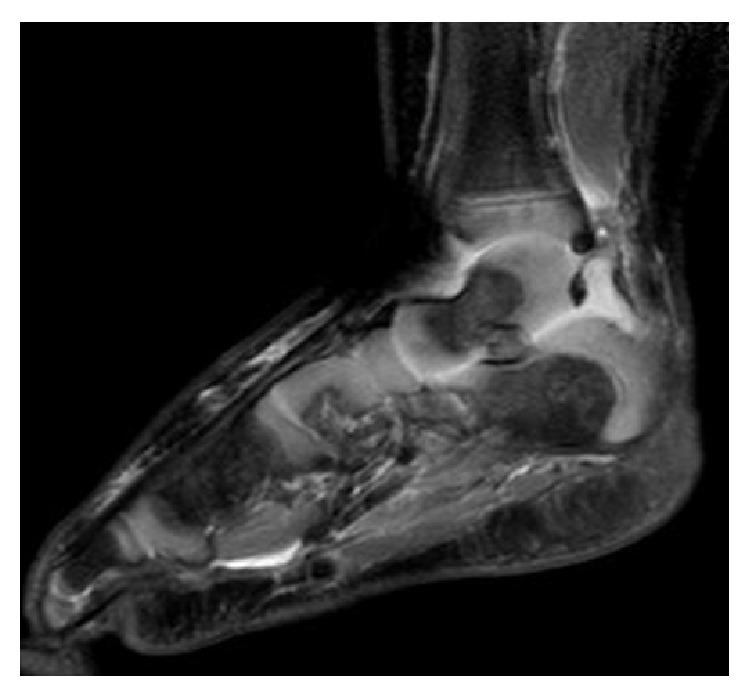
MRI of right ankle reveals hyperintense fluid in the tibiotalar and the subtalar joint with no signs of osteomyelitis.
